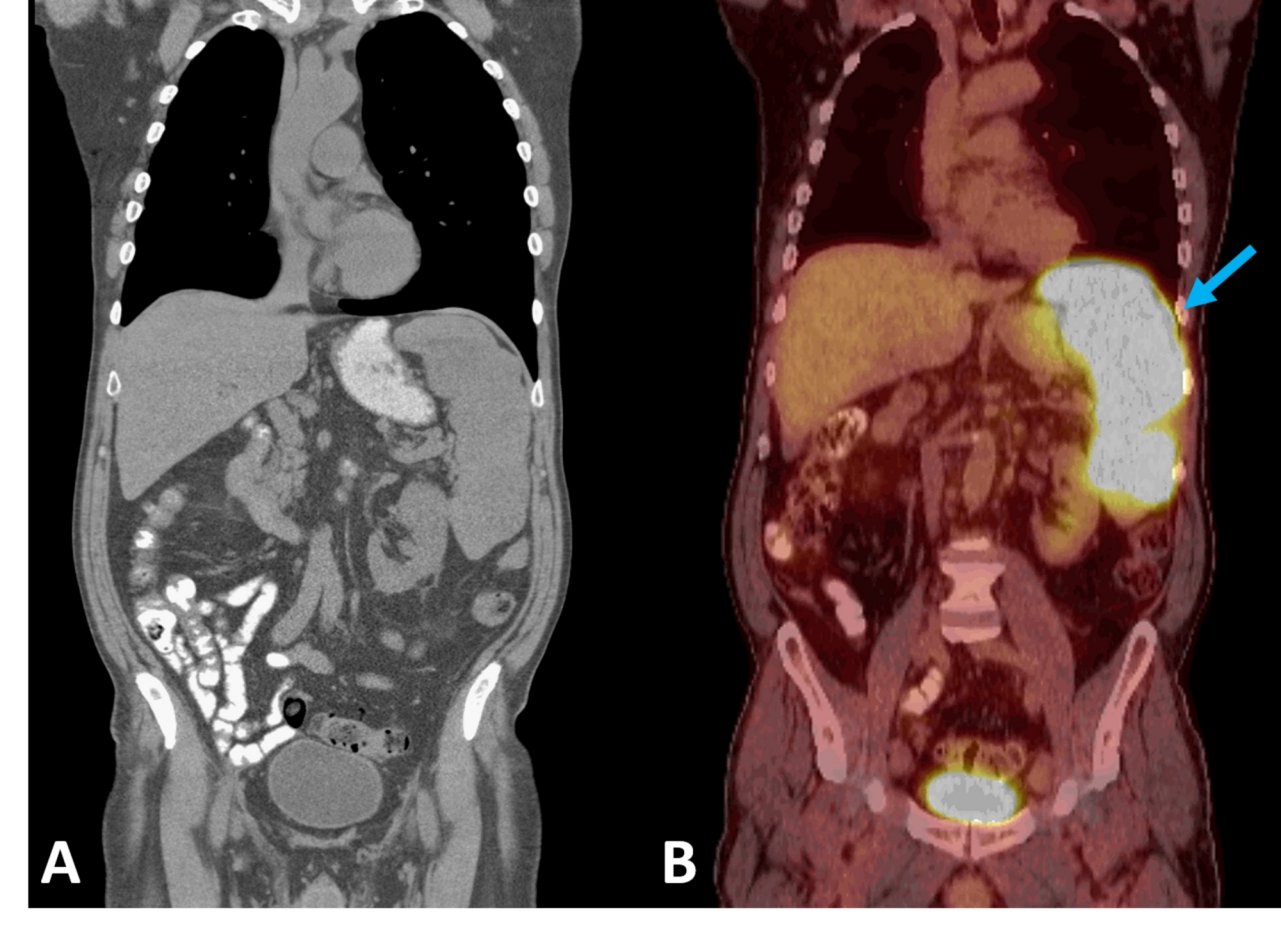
Left (A): CT of the chest, abdomen, pelvis without contrast read as: “No malignancy within the chest, abdomen, or pelvis, nonspecific splenomegaly, and calcified pleural plaques.” Right (B): Nuclear medicine PET tumor scan read as: “Splenomegaly with markedly hypermetabolic large foci of hypermetabolic activity, concerning for lymphoma (arrow). No additional or extra-nodal disease is seen in the chest, abdomen, and pelvis.” CT: computed tomography; PET: positron emission tomography
